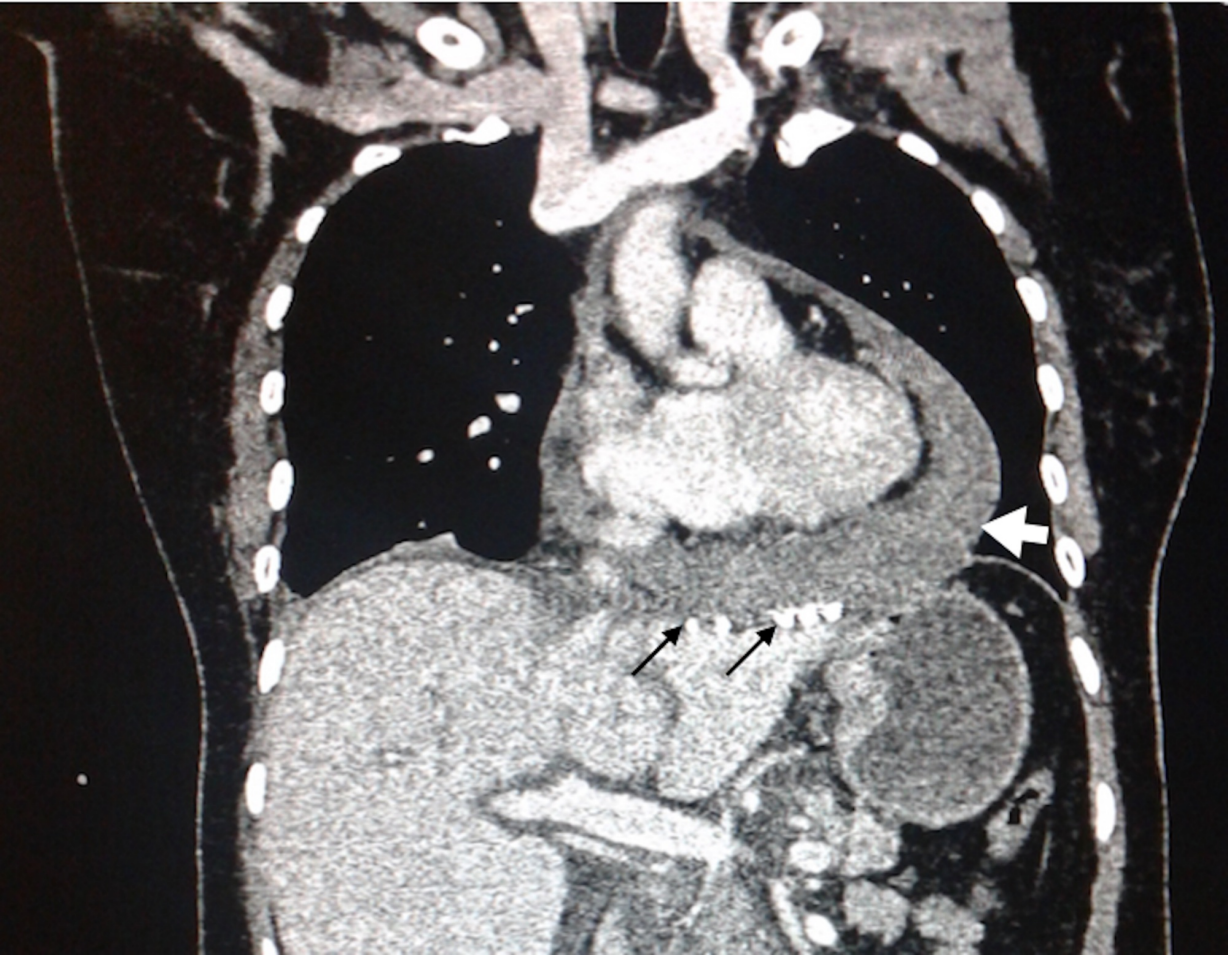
CT scan, coronal plane. Close approximation of tacks to the pericardium (thin black arrows) causing pericardial effusion (thick white arrow).
